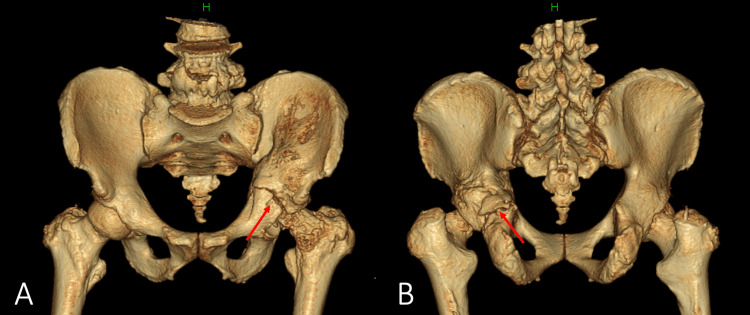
3-D CT of the pelvis at our hospital Pseudarthrosis of the transverse-posterior wall acetabular fracture and destruction of the femoral head have been demonstrated (red arrows)
